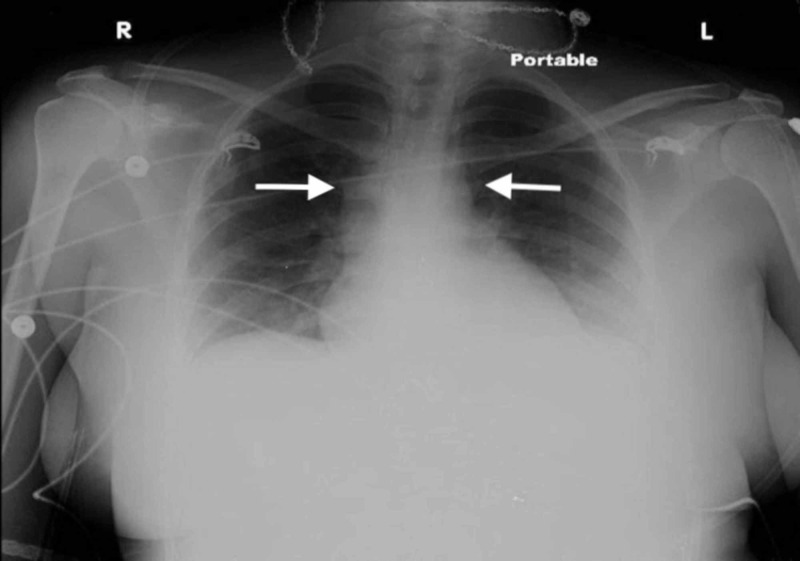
Chest X-ray showing mediastinal lymphadenopathy (white arrows) and bilateral pleural effusions.
